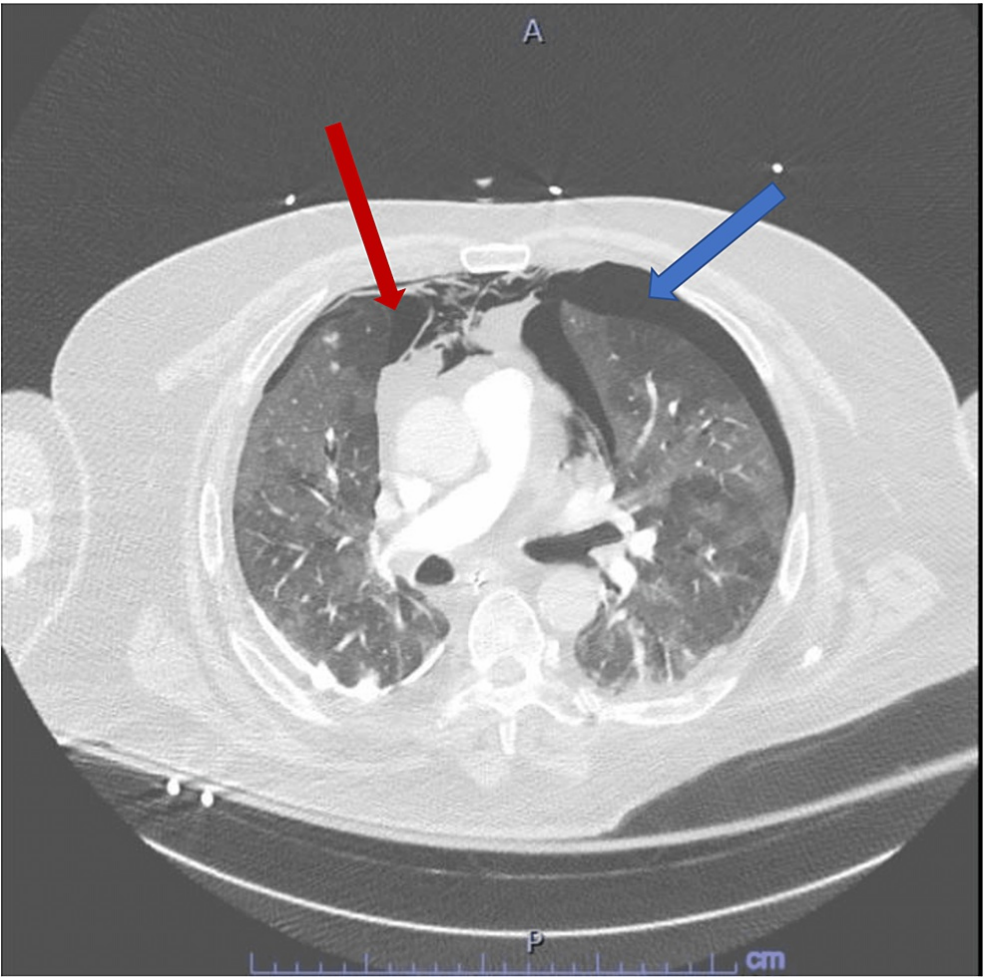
CTPA, cross-sectional view, lung window. Blue arrow left side pneumothorax, red arrow right side pneumothorax. CTPA, CT pulmonary angiogram
